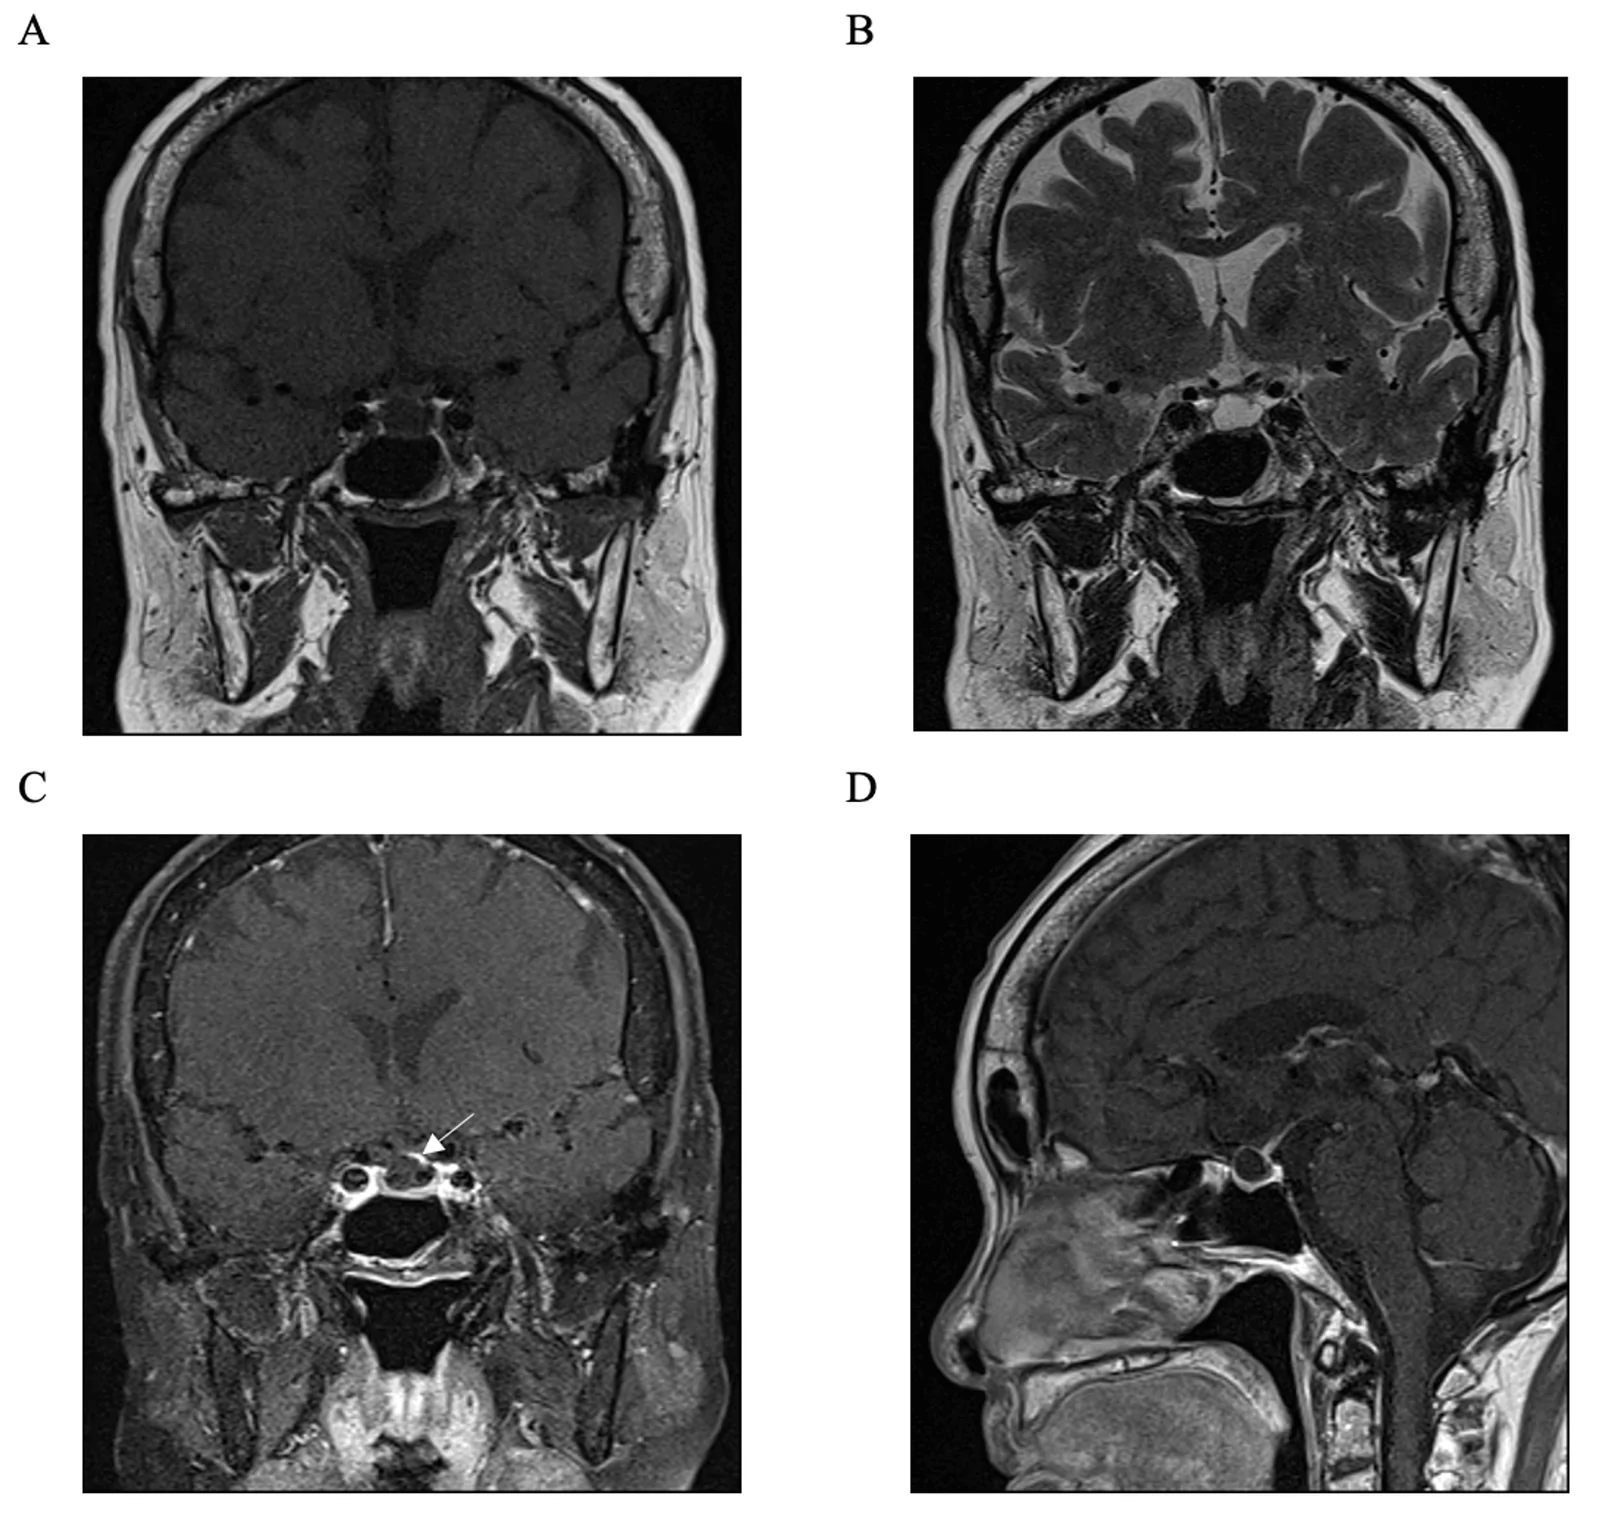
Magnetic resonance imaging (MRI) sequences, including coronal T1-weighted (A), coronal T2-weighted (B), and post-contrast images in coronal and sagittal (C, D) demonstrate a Rathke’s cleft cyst causing mild compression and leftward displacement of the pituitary stalk (arrow).
